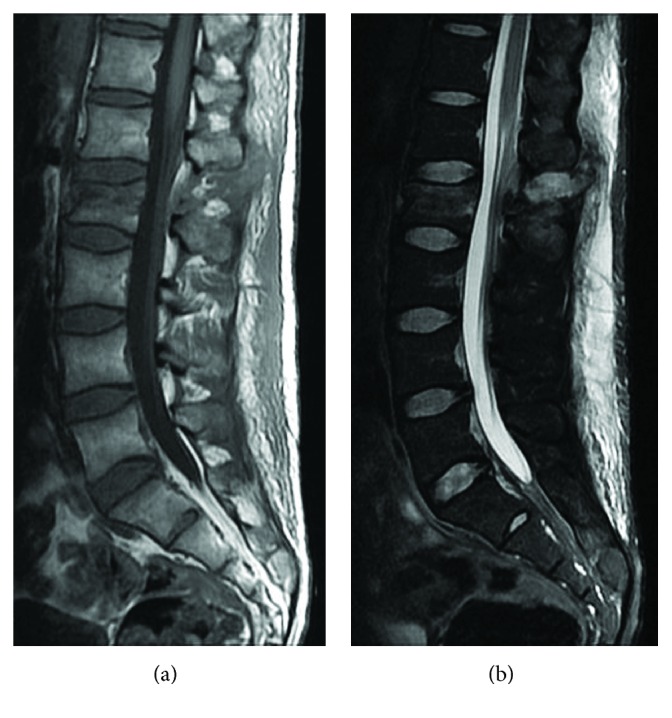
Preoperative magnetic resonance imaging (MRI) of the lumbar spine. (a) Midsagittal T1-weighted and (b) short tau inversion and recovery (STIR) MRI showing the L2 vertebral fracture and disruption of the posterior ligamentous complex between L1 and L2 in combination with extensive subcutaneous hematoma.
